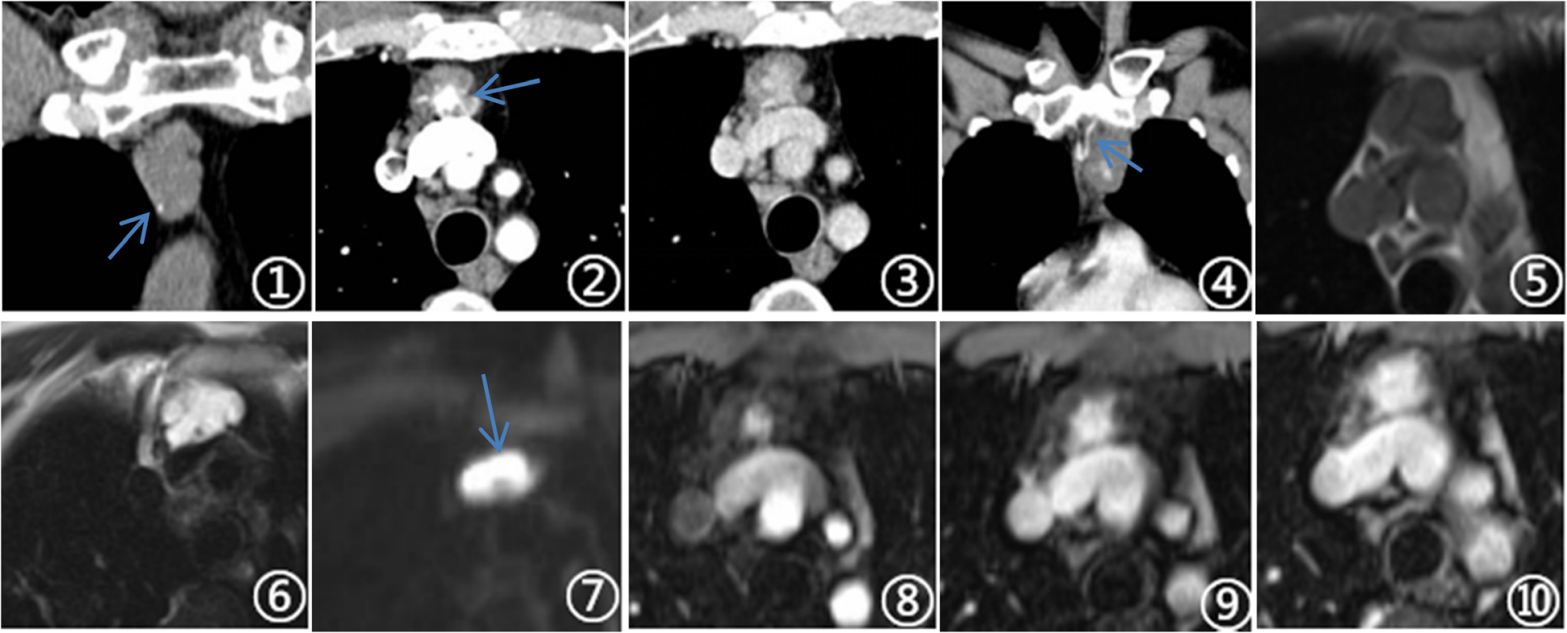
1-10 were the same case. 1 Lesion has equal density and small circular calcifications were observed inside. 6-7 Mass on the fat suppression and DWI (*b* = 800) showed obviously high signal with small blood vessel shadows inside. 2-3 and 7-10 In the arterial phase, in the center of the lesions, there is the nodular enhancement. As time goes by, the enhanced range was expanded and showed a “fast in and slow out” performance. 4, 8 In the arterial phase, aberrant draining veins filled by the contrast agent antidromic could be observed, which were connected to the left brachiocephalic vein
